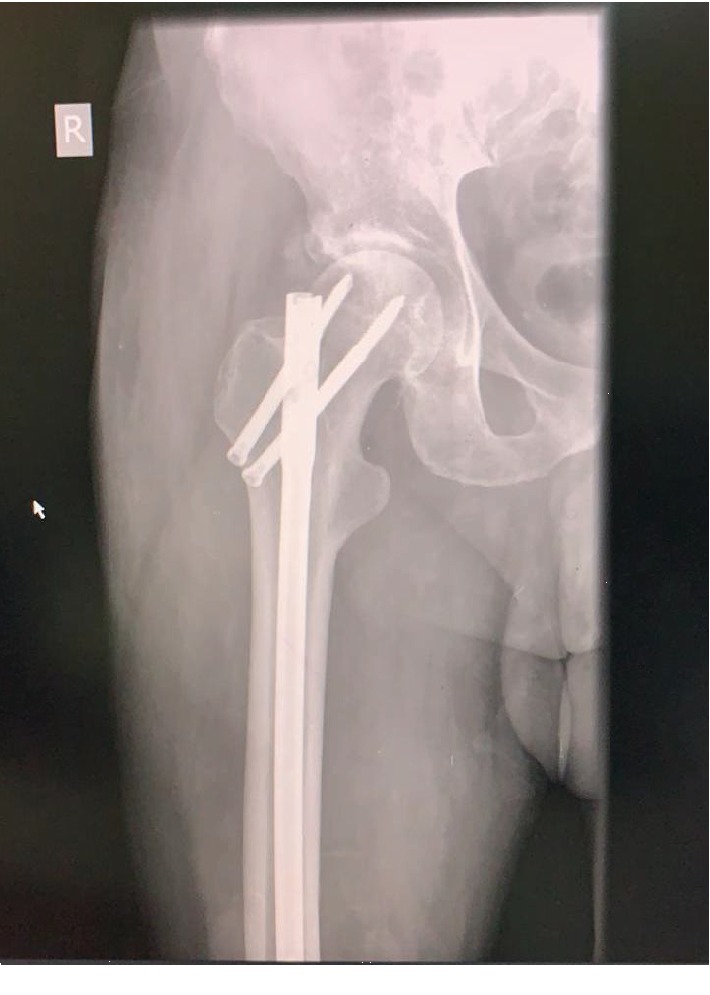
Radiograph shows femur with proximal locking in AP view.
